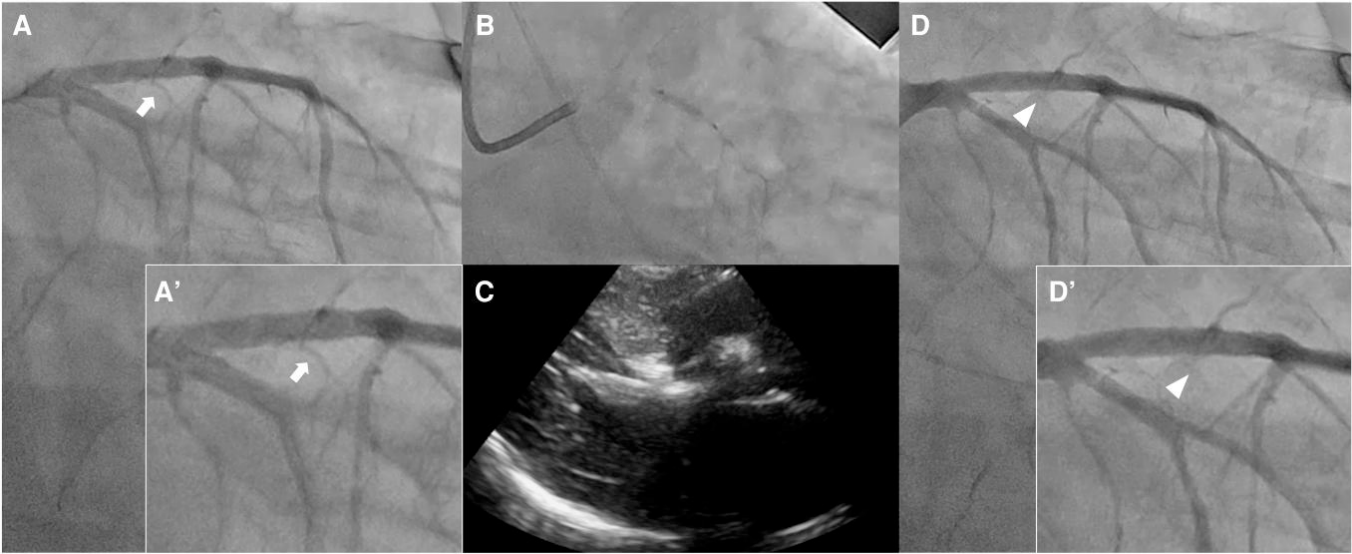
Percutaneous transluminal septal myocardial ablation (PTSMA) procedure. *(A)* First angiography of the left coronary artery with right anterior oblique-caudal view. White arrows indicate the target septal branches. *(A)* Magnified view of the same area. *(B)* Tip injection from the overwire balloon. *(C)* Septal myocardial opacification on echocardiography. *(D)* Final angiography of the left coronary artery with right anterior oblique-caudal view. White arrowheads indicate the disappearance of the target septal branch. d: magnified view of the same area.
